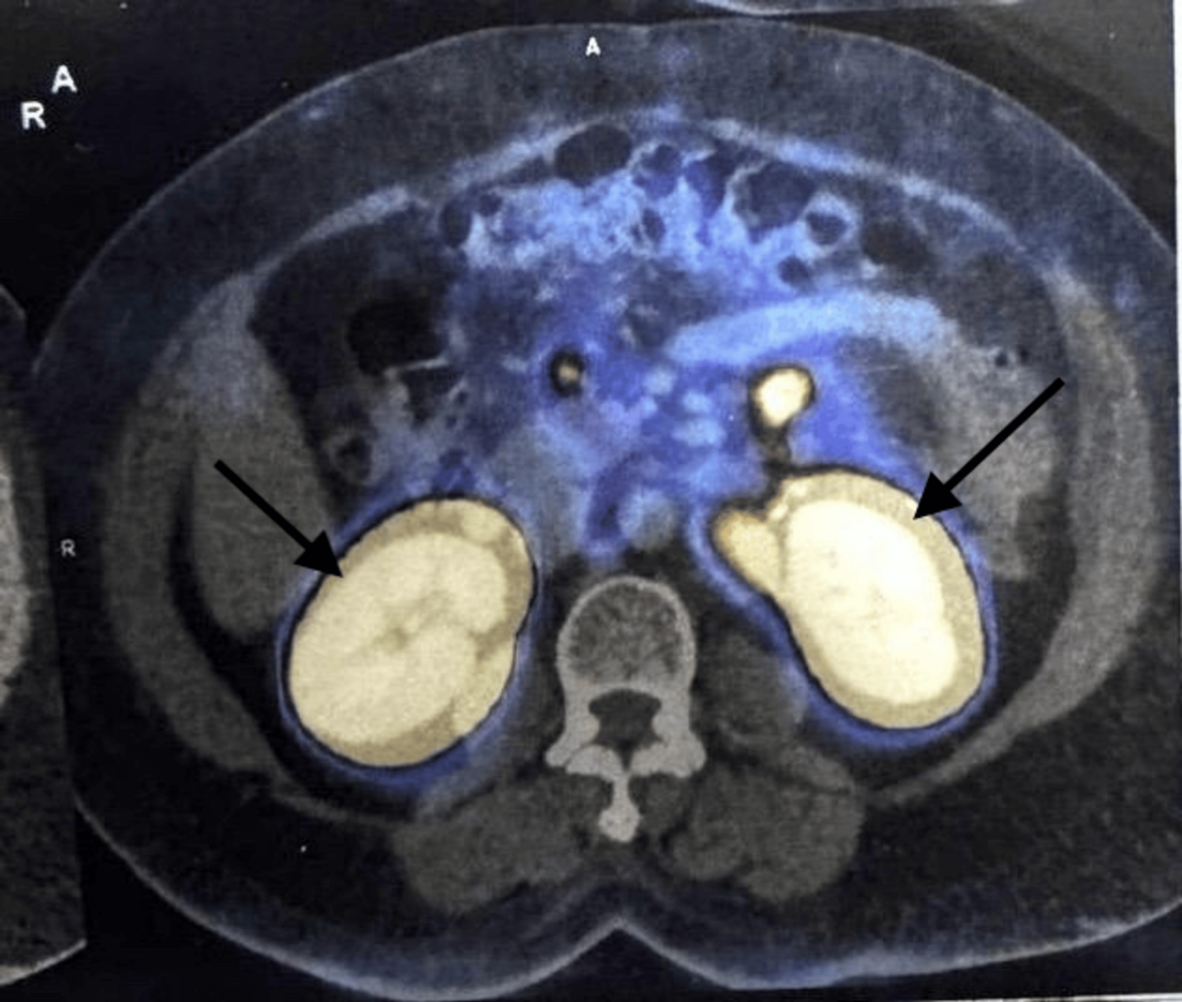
Ga-68 DOTA-exendin positron emission tomography-computed tomography (PET-CT) with a tracer avid exophytic lesion involving the neck of the pancreas
